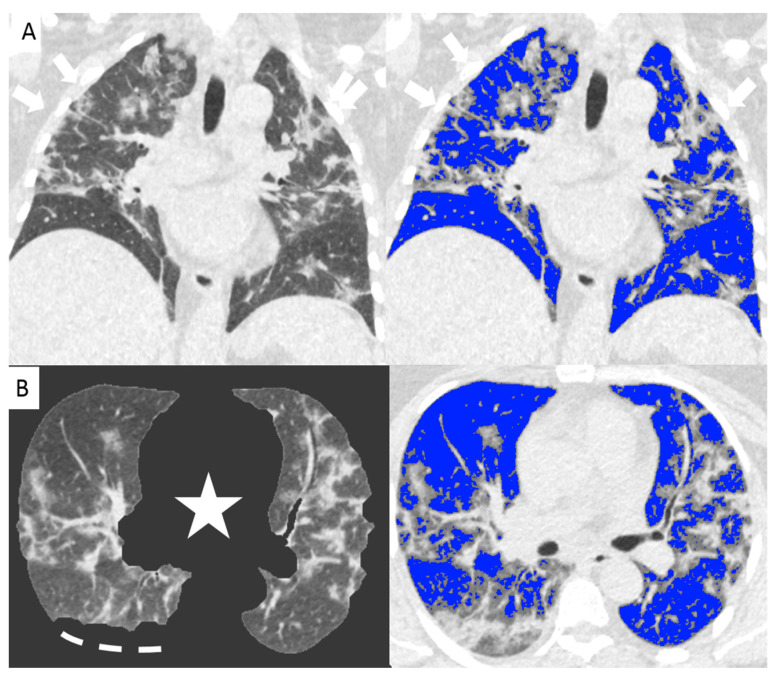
Non-enhanced coronal reconstructions of Chest CT (A), (upper panel) showing bilateral ground glass opacities with random distribution and consolidations in the upper and lower lobes (arrows). Lower panel show axial CT reconstructions of the same patient with automatic software segmentation (B) excluding all “non-lung” structures, (star) and not evaluable parenchyma due to pleural effusion (segmented line). Images on the right show software evaluation of the well aerated lung (blue) and the infected parenchyma (grey zone, arrows) discriminated by specific threshold. The whole infected volume assessed by software was 47% of the entire lung volume. The patient underwent invasive ventilation.
